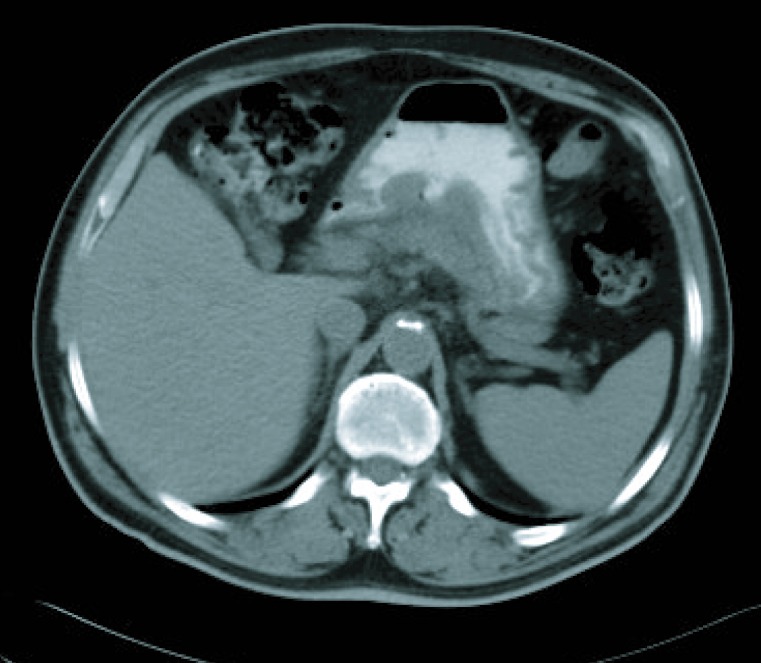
CT imaging after 6 cicles.
